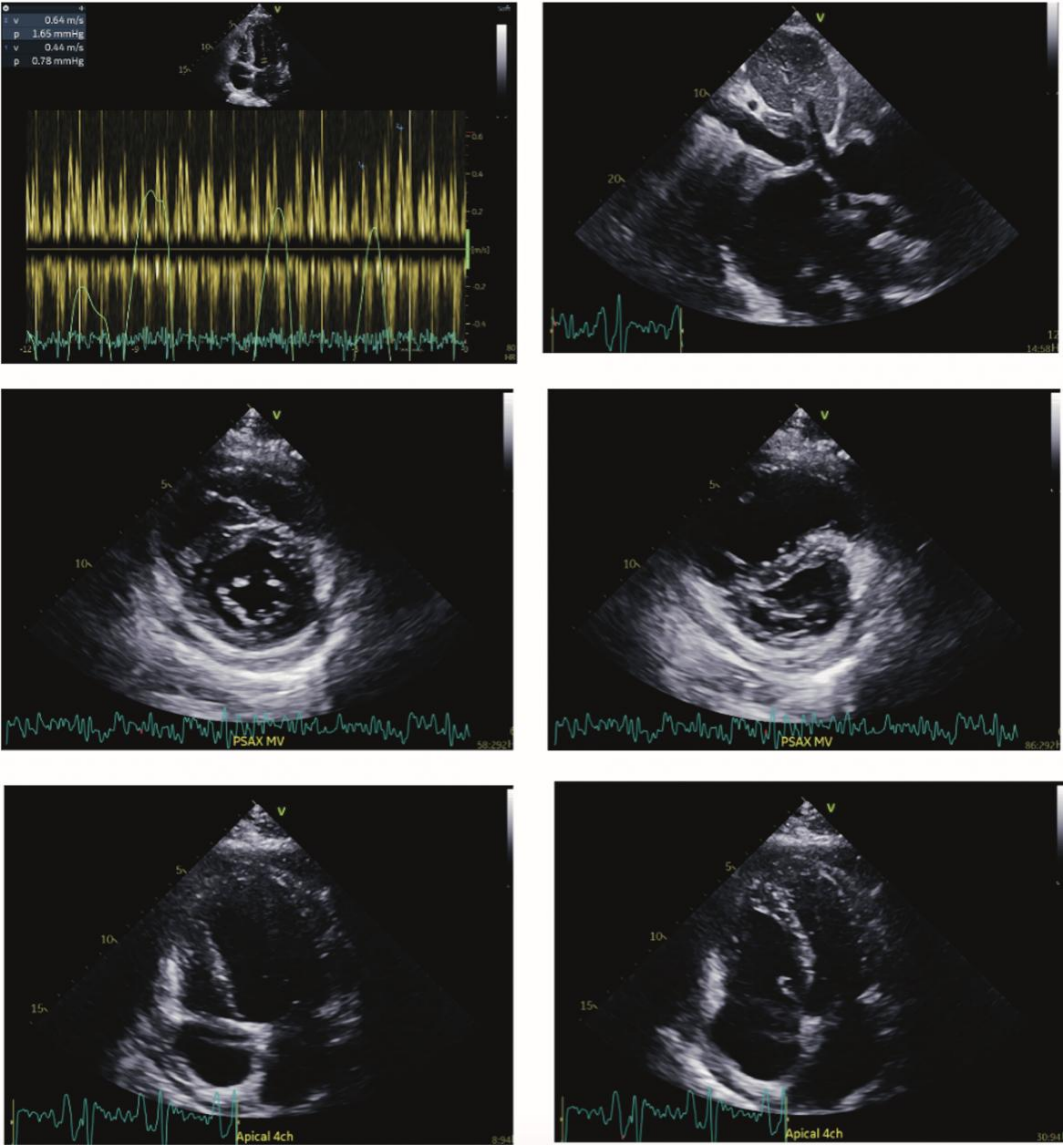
Index transthoracic echocardiogram demonstrating mitral valve inflow variation (*A*), inferior vena cava dilatation (*B*), and septal bounce (*C–F*).
